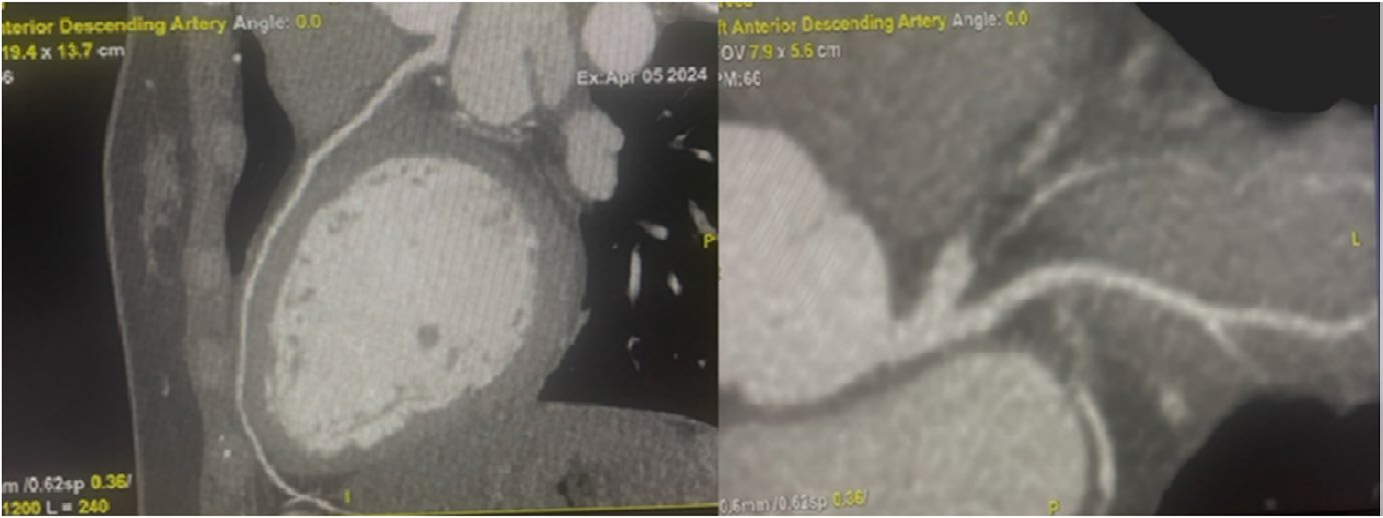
CT angiography of the patient showing unremarkable anatomical variation and nonobstructive coronaries.
